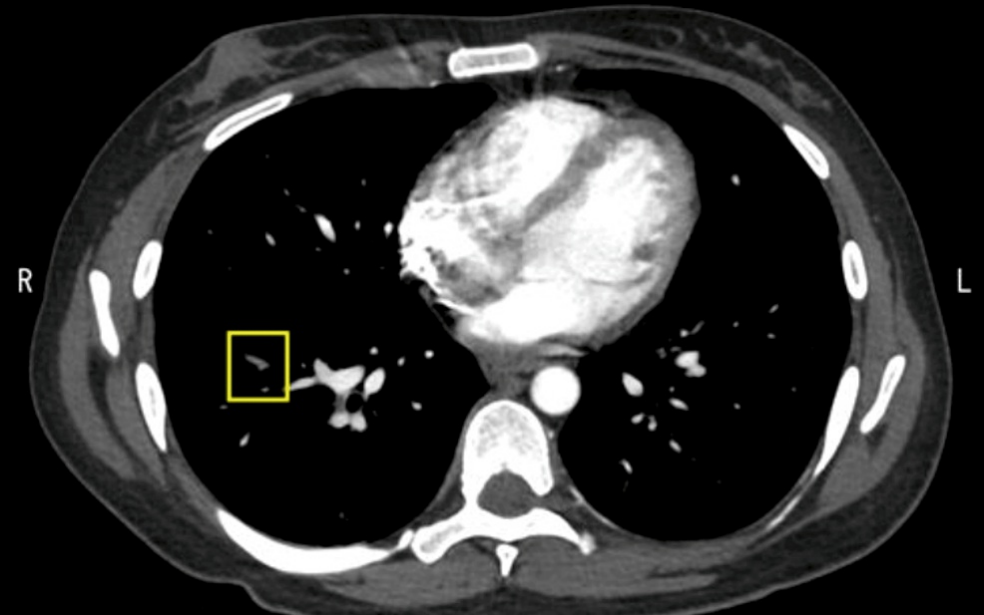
Initial imaging study of the chest An enhanced CT image of the chest on initial presentation shows right pulmonary embolisms (framed areas).
